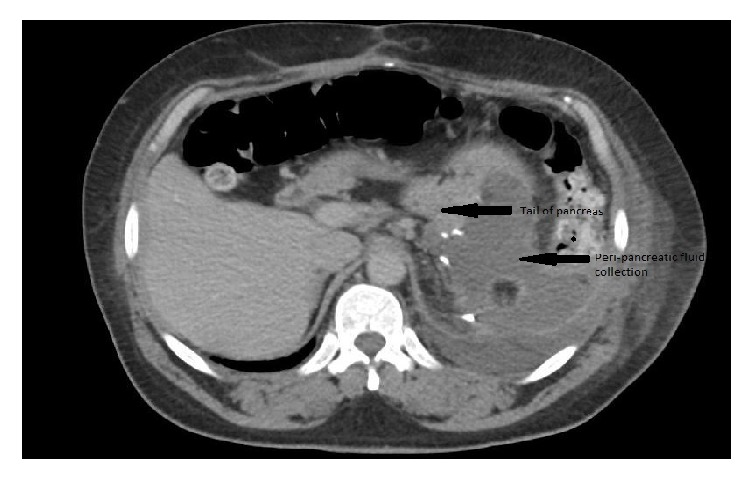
Patient 2, CT scan abdomen showing fluid collection around the tail of pancreas.
